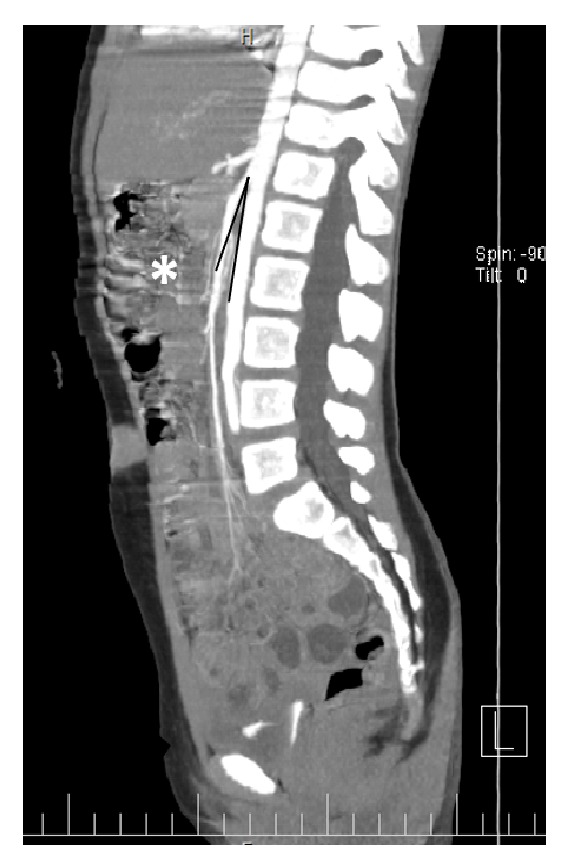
Contrast-enhanced sagittal reconstruction CT scan of superior mesenteric artery (SMA) and its adjacent anatomy shows narrowing of the aortomesenteric angle to less than 15° and distended stomach (*∗*) and full of fluids loops of the small intestine.
